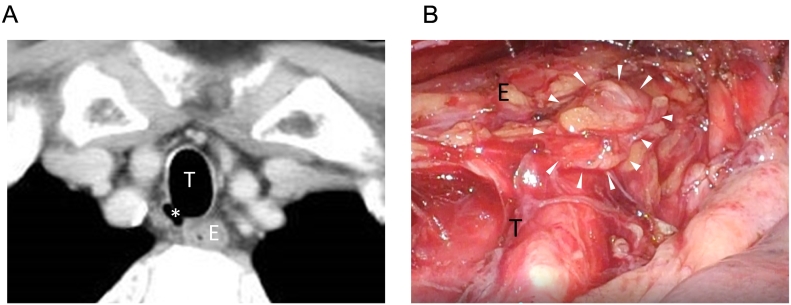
(A) Preoperative CT image of the PTAC in Patient 1 (asterisk). (B) Intraoperative findings of PTAC in Patient 1 (white arrow heads). E: esophagus, T: trachea.
